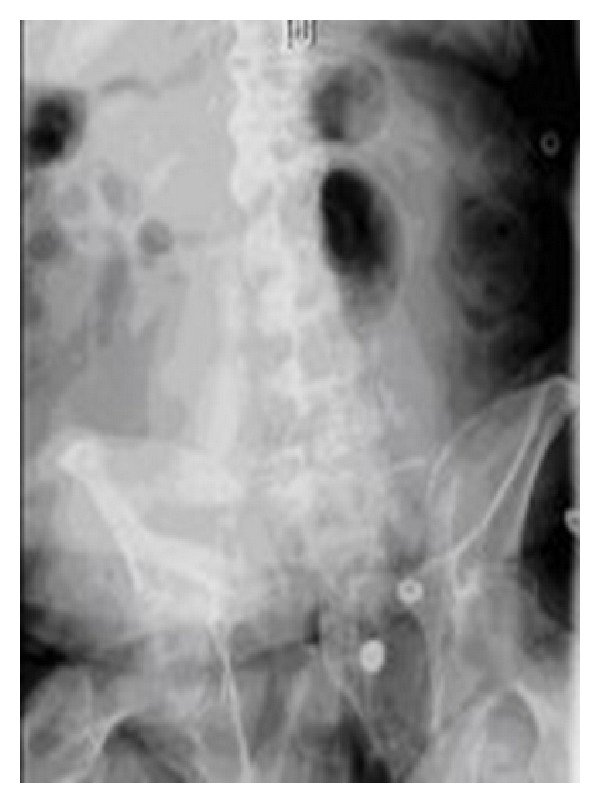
The second abdominal X-ray, performed after the neuroleptic was stopped the second time leading to the disappearance of the digestive symptoms and the return to a normal stomach size.
